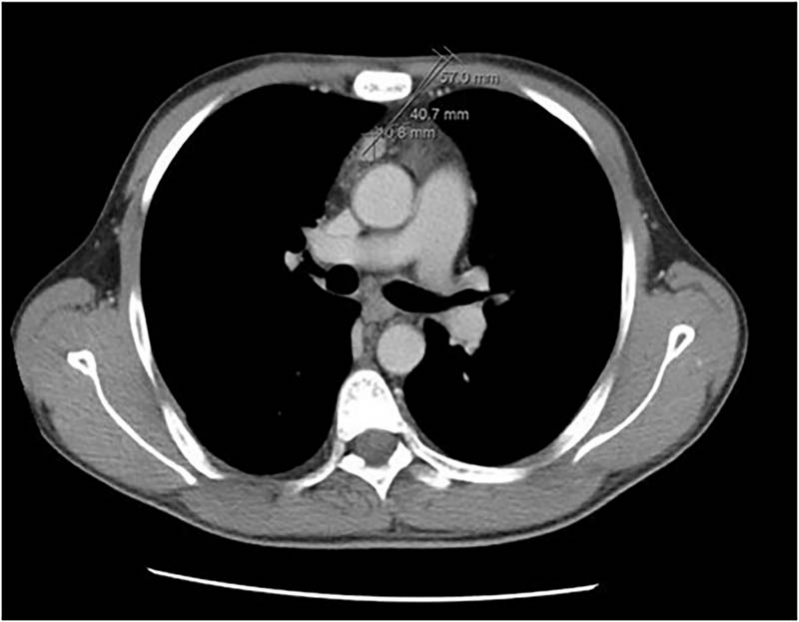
Chest CT revealing a retrosternal lesion measuring 57 mm anterior to the ascending aorta.
